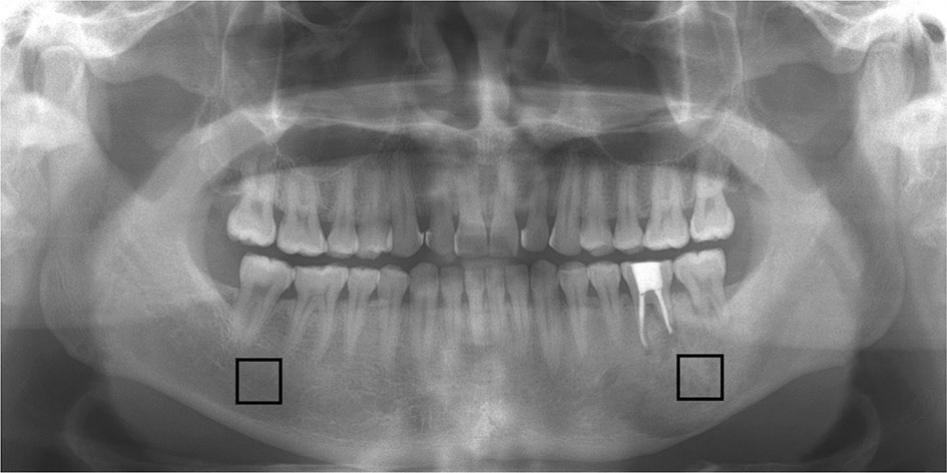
Panoramic radiograph of a 48-year-old man with osteosarcoma (OS) on the left mandible. Note the location of the two square regions of interest (ROIs): one ROI at the center of the OS on the left mandibular body and the other ROI in the corresponding normal trabecular bone on the right mandibular body.
